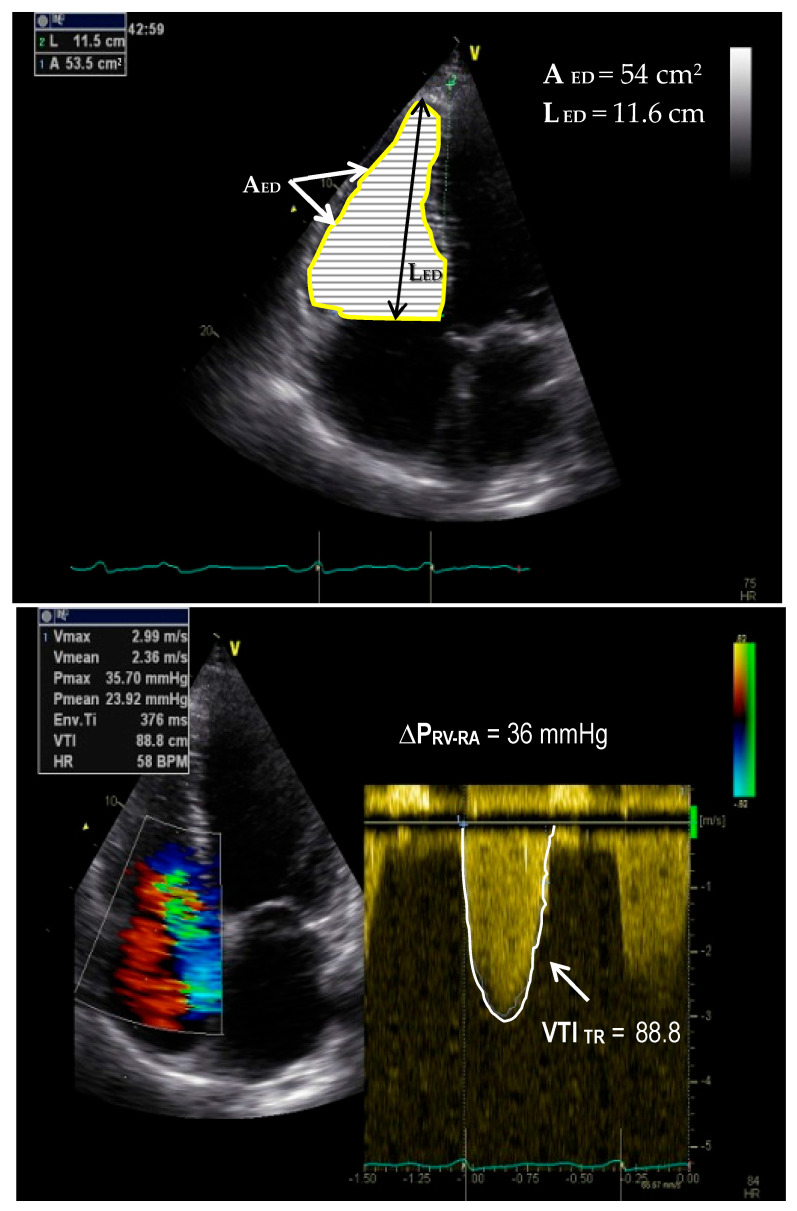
Calculation of the RV load-adaptation index from 2D-ECHO-derived measurements [11,15,20,32,60,149,226,227,228,229,230,234,235]. Upper image: Measurement of RV end-diastolic area and long-axis lengths (A_ED_ and L_ED_, respectively). Lower image: Measurement of the velocity–time integral (VTI _RV_) by Doppler-ECHO. Based on those measurements, the LAI_RV_ calculation results in a value of 19, which indicates a reduced RV adaptability to increased afterload, but with a still potential reversibility by reduction of its hemodynamic overloading.
